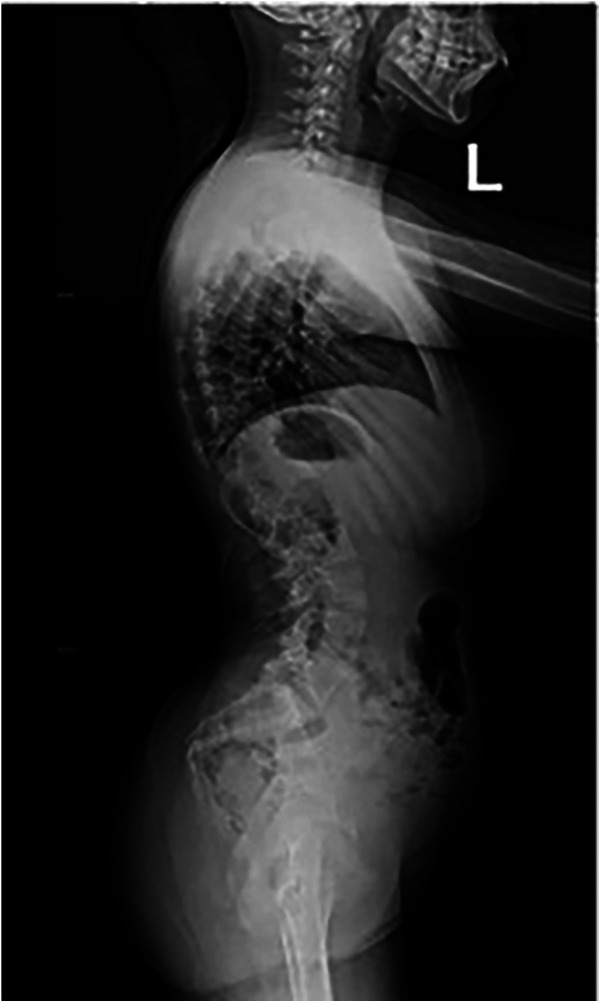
Preoperative lateral radiograph of the spine demonstrating thoracolumbar deformity and pelvic obliquity in a patient with neuromuscular scoliosis.
